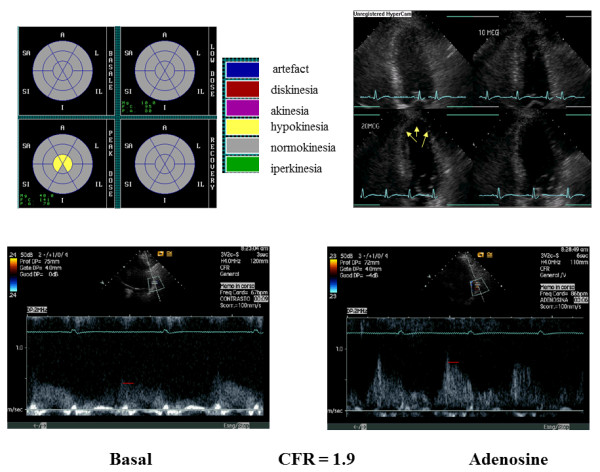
Doppler echocardiography image of a SSc patient with WMA and impaired CFR. Doppler echocardiography image of a patient with wall motion abnormalities (hypokinesia of apical segments) during Dobutamine Stress Echocardiography (upper panel) and abnormal Coronary Flow Reserve (lower pannel).
